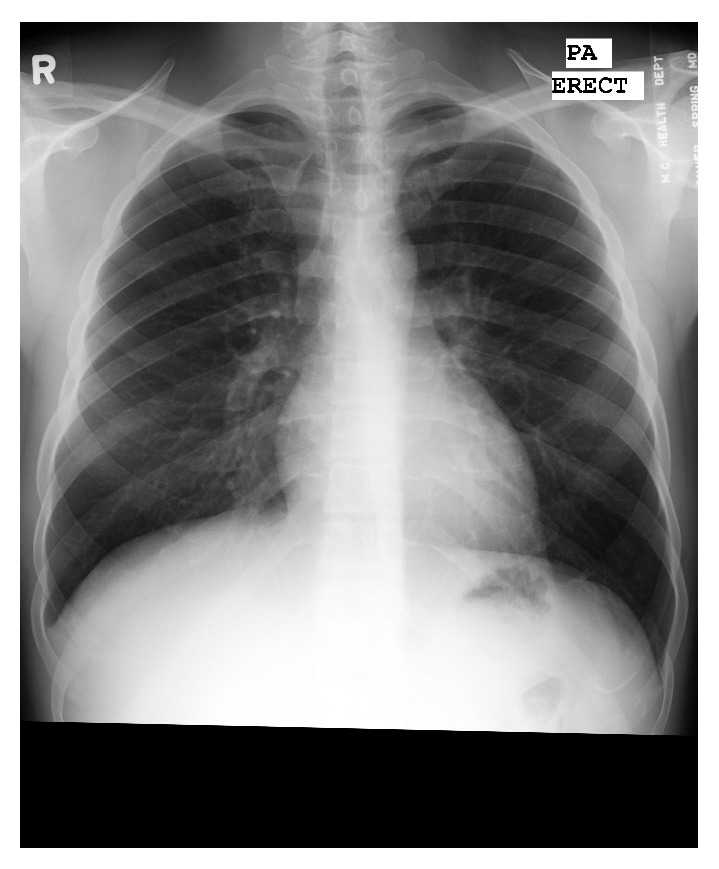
Normal chest X-ray (CXR).
